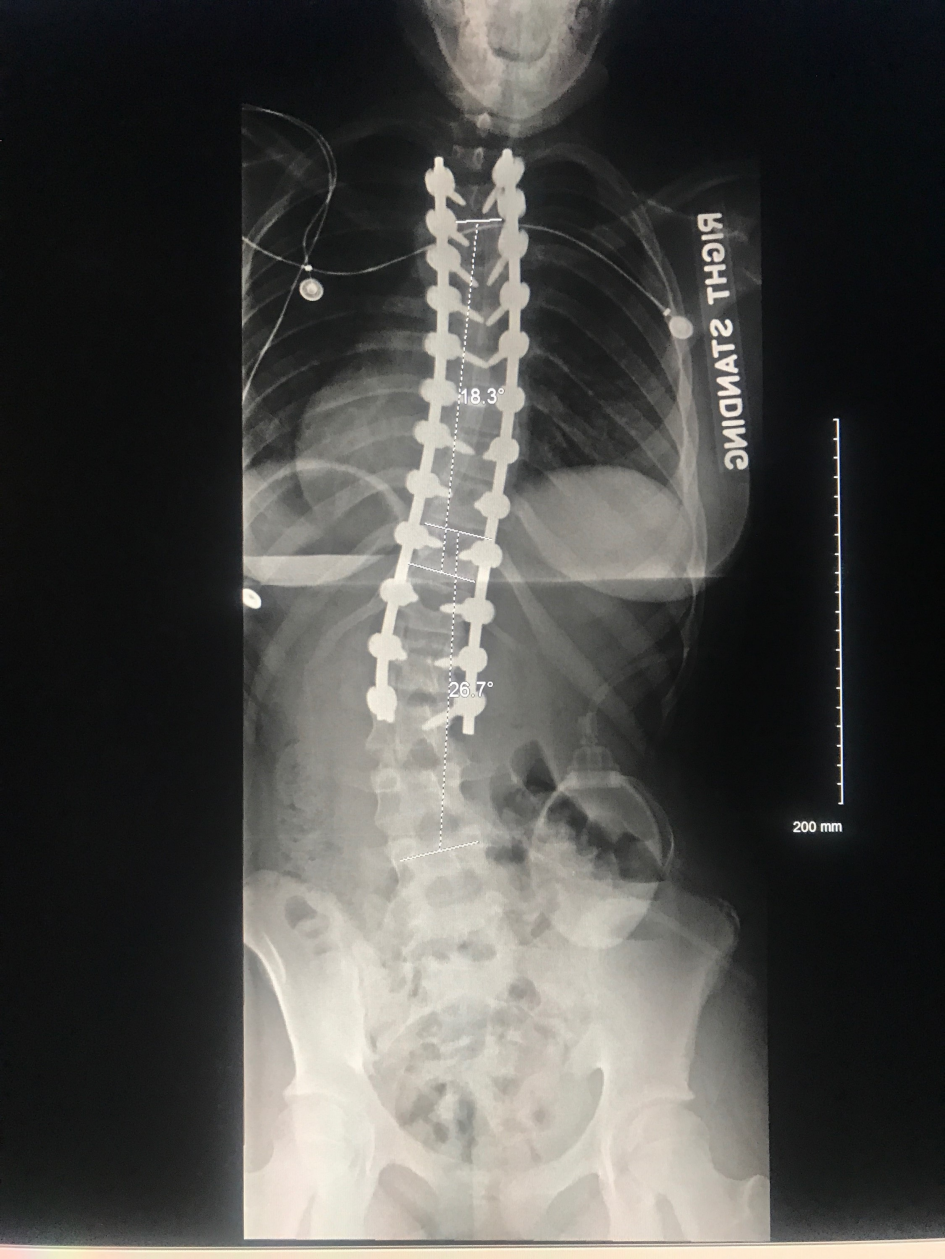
Postoperative posteroanterior (PA) 36-inch standing scoliosis radiograph.
